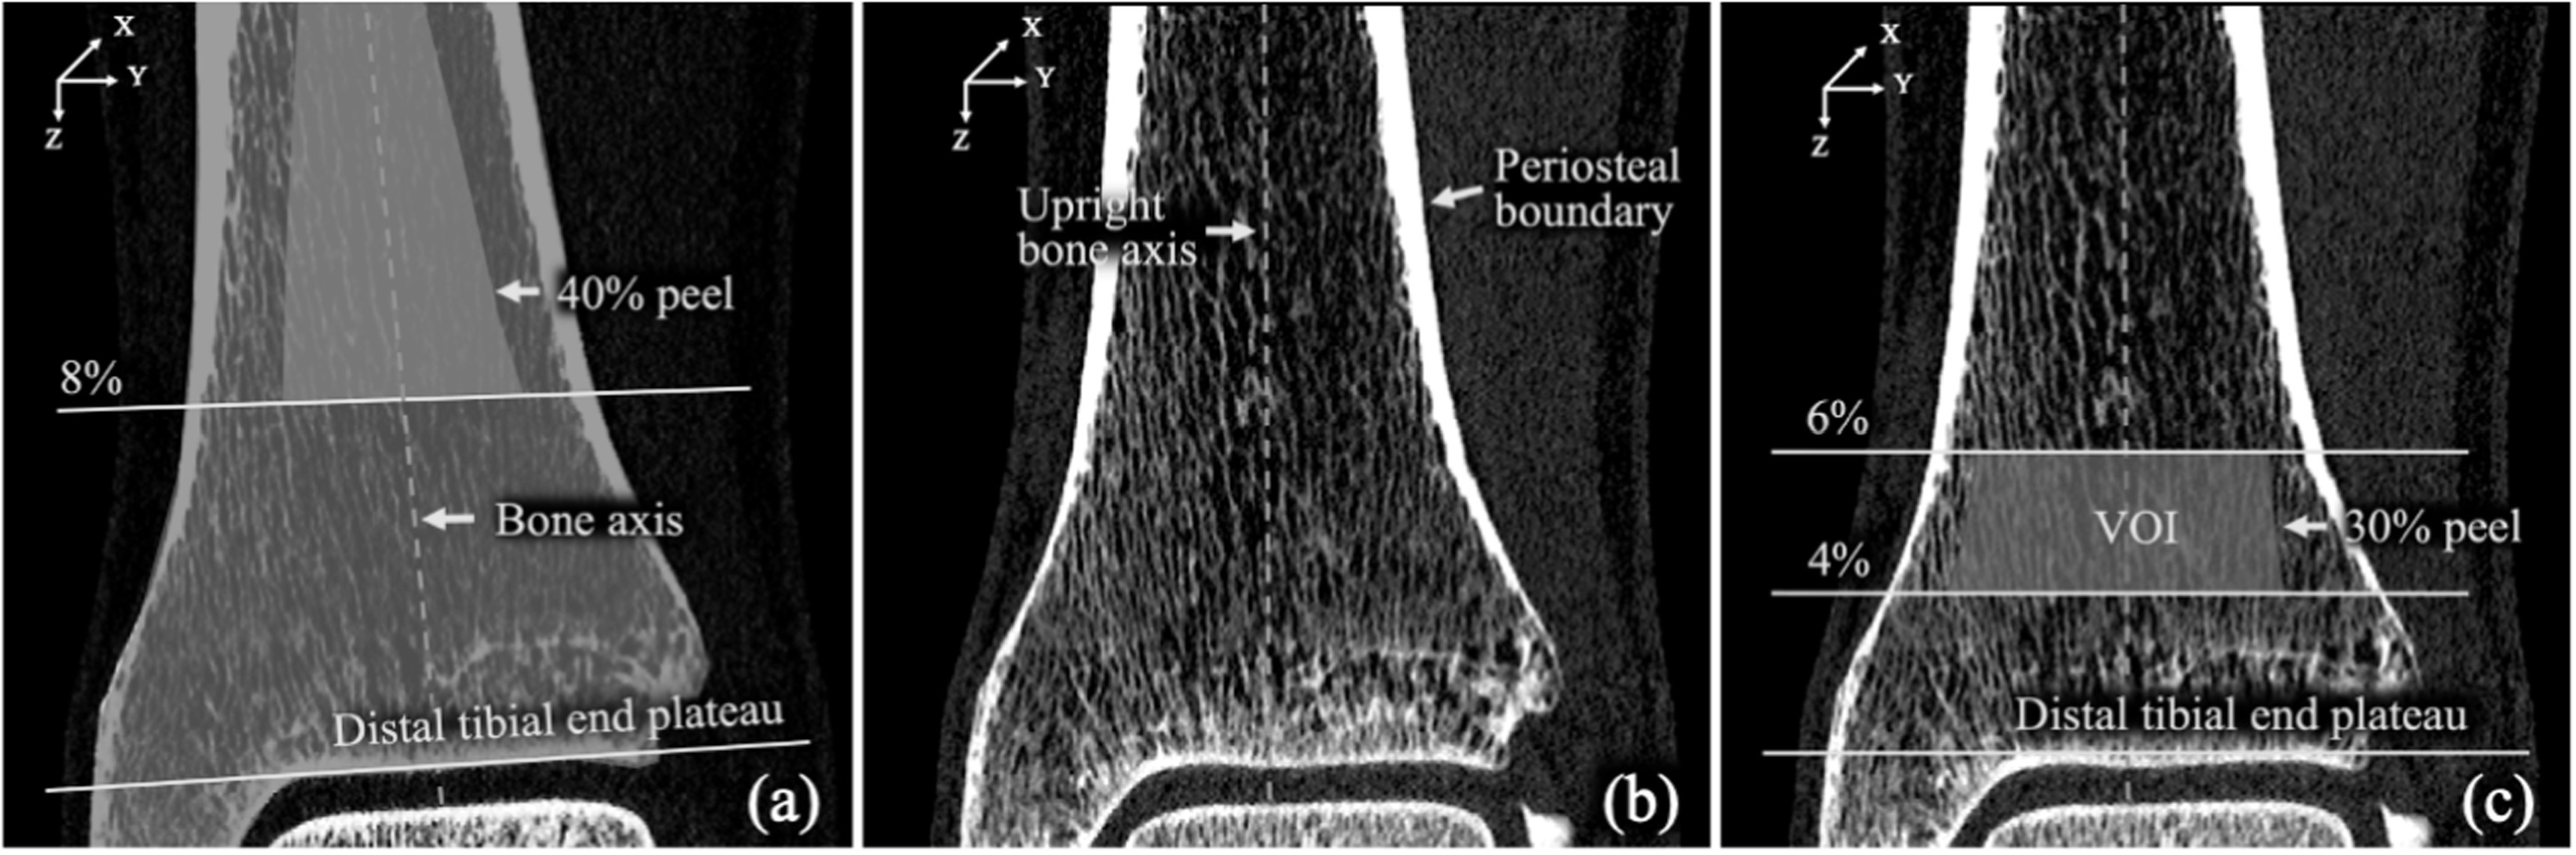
Bone alignment and VOI selection on a distal tibia CT scan for FEA. (a) Segmented tibial bone region (green) on a sagittal image slice and quasi-cylindrical region (magenta) for bone axis computation. (b) A sagittal CT slice after alignment of the tibial bone axis. (c) Selected VOI (blue) for FEA.
